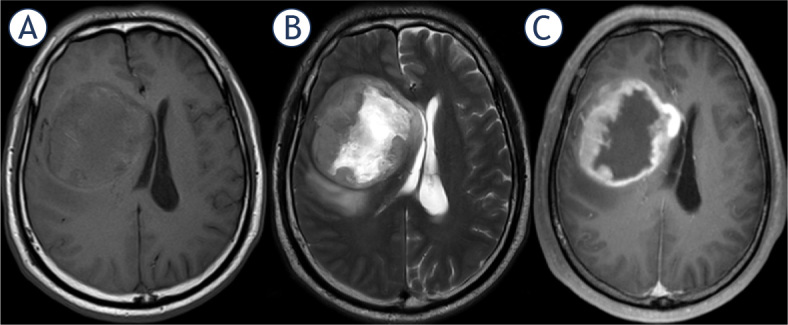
Representative MR images with imaging necrosis derived from a 53-year-old man with glioblastoma, IDH-wildtype. Shown from left to right by the order are T1WI (A), T2WI (B), and T1WI-CE (C).
